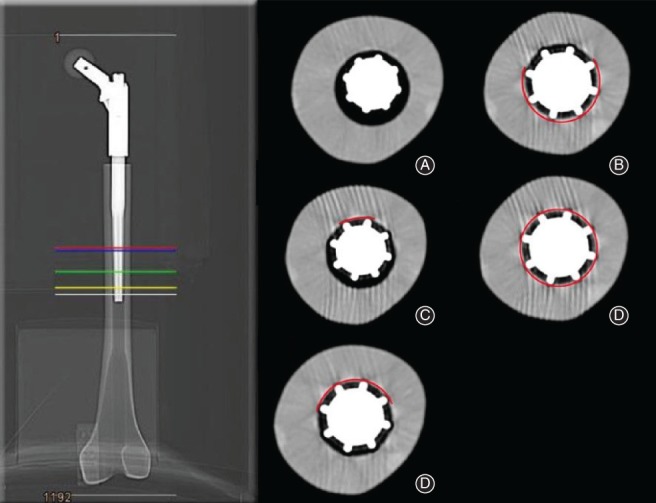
Analysis of the metal–bone interface with cross‐section references of the stem using a CT scan; (A) white line with 0% stem–bone interface, (B) yellow line with 25% stem–bone interface, (C) green line with 50% stem–bone interface, (D) blue line with 75% stem–bone interface and (E) red line with 100% stem–bone interface.
